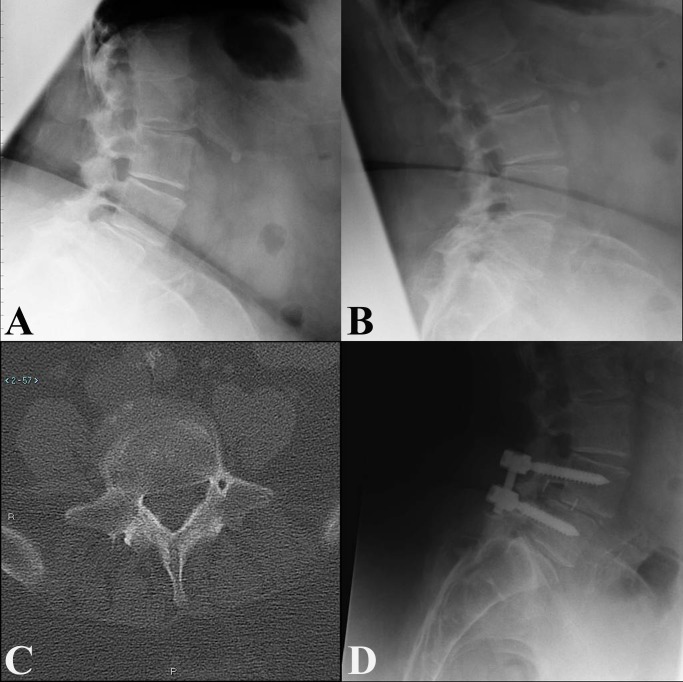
Interbody Graft Extrusion in a Unilaterally-Fixated Patient with an Abnormal Pedicle (A, B) Preoperative flexion (A) and extension (B) radiographs demonstrating anterolisthesis which increases >3mm in flexion. (C) Axial computed tomography through L5 demonstrating a vascular or neural structure within the left L5 pedicle.  (D) 3-month follow-up lateral radiograph demonstrating posterior graft migration.
